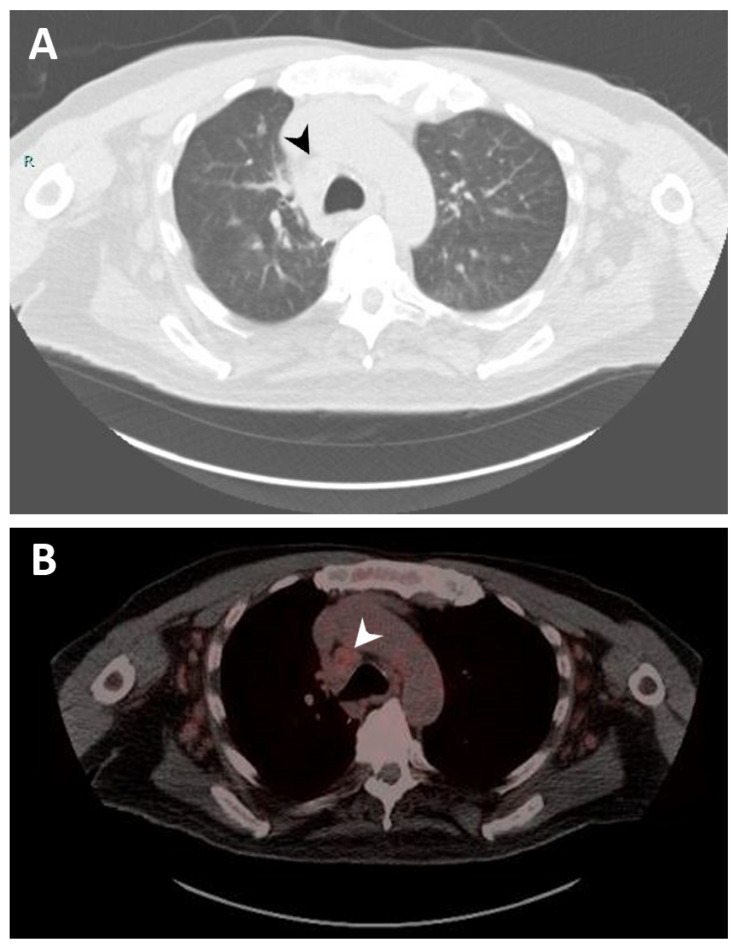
Imaging studies. (A) Computerized tomography (CT) scan of the chest showing right lower paratracheal lymphadenopathy measuring 2.1 cm (black arrow). (B) Gallium-68 dotatate positron emission tomography (PET)/CT scan of the chest demonstrating right lower paratracheal lymphadenopathy with mild radiotracer uptake, suggesting a neoplastic process related to mantle cell lymphoma (white arrow).
